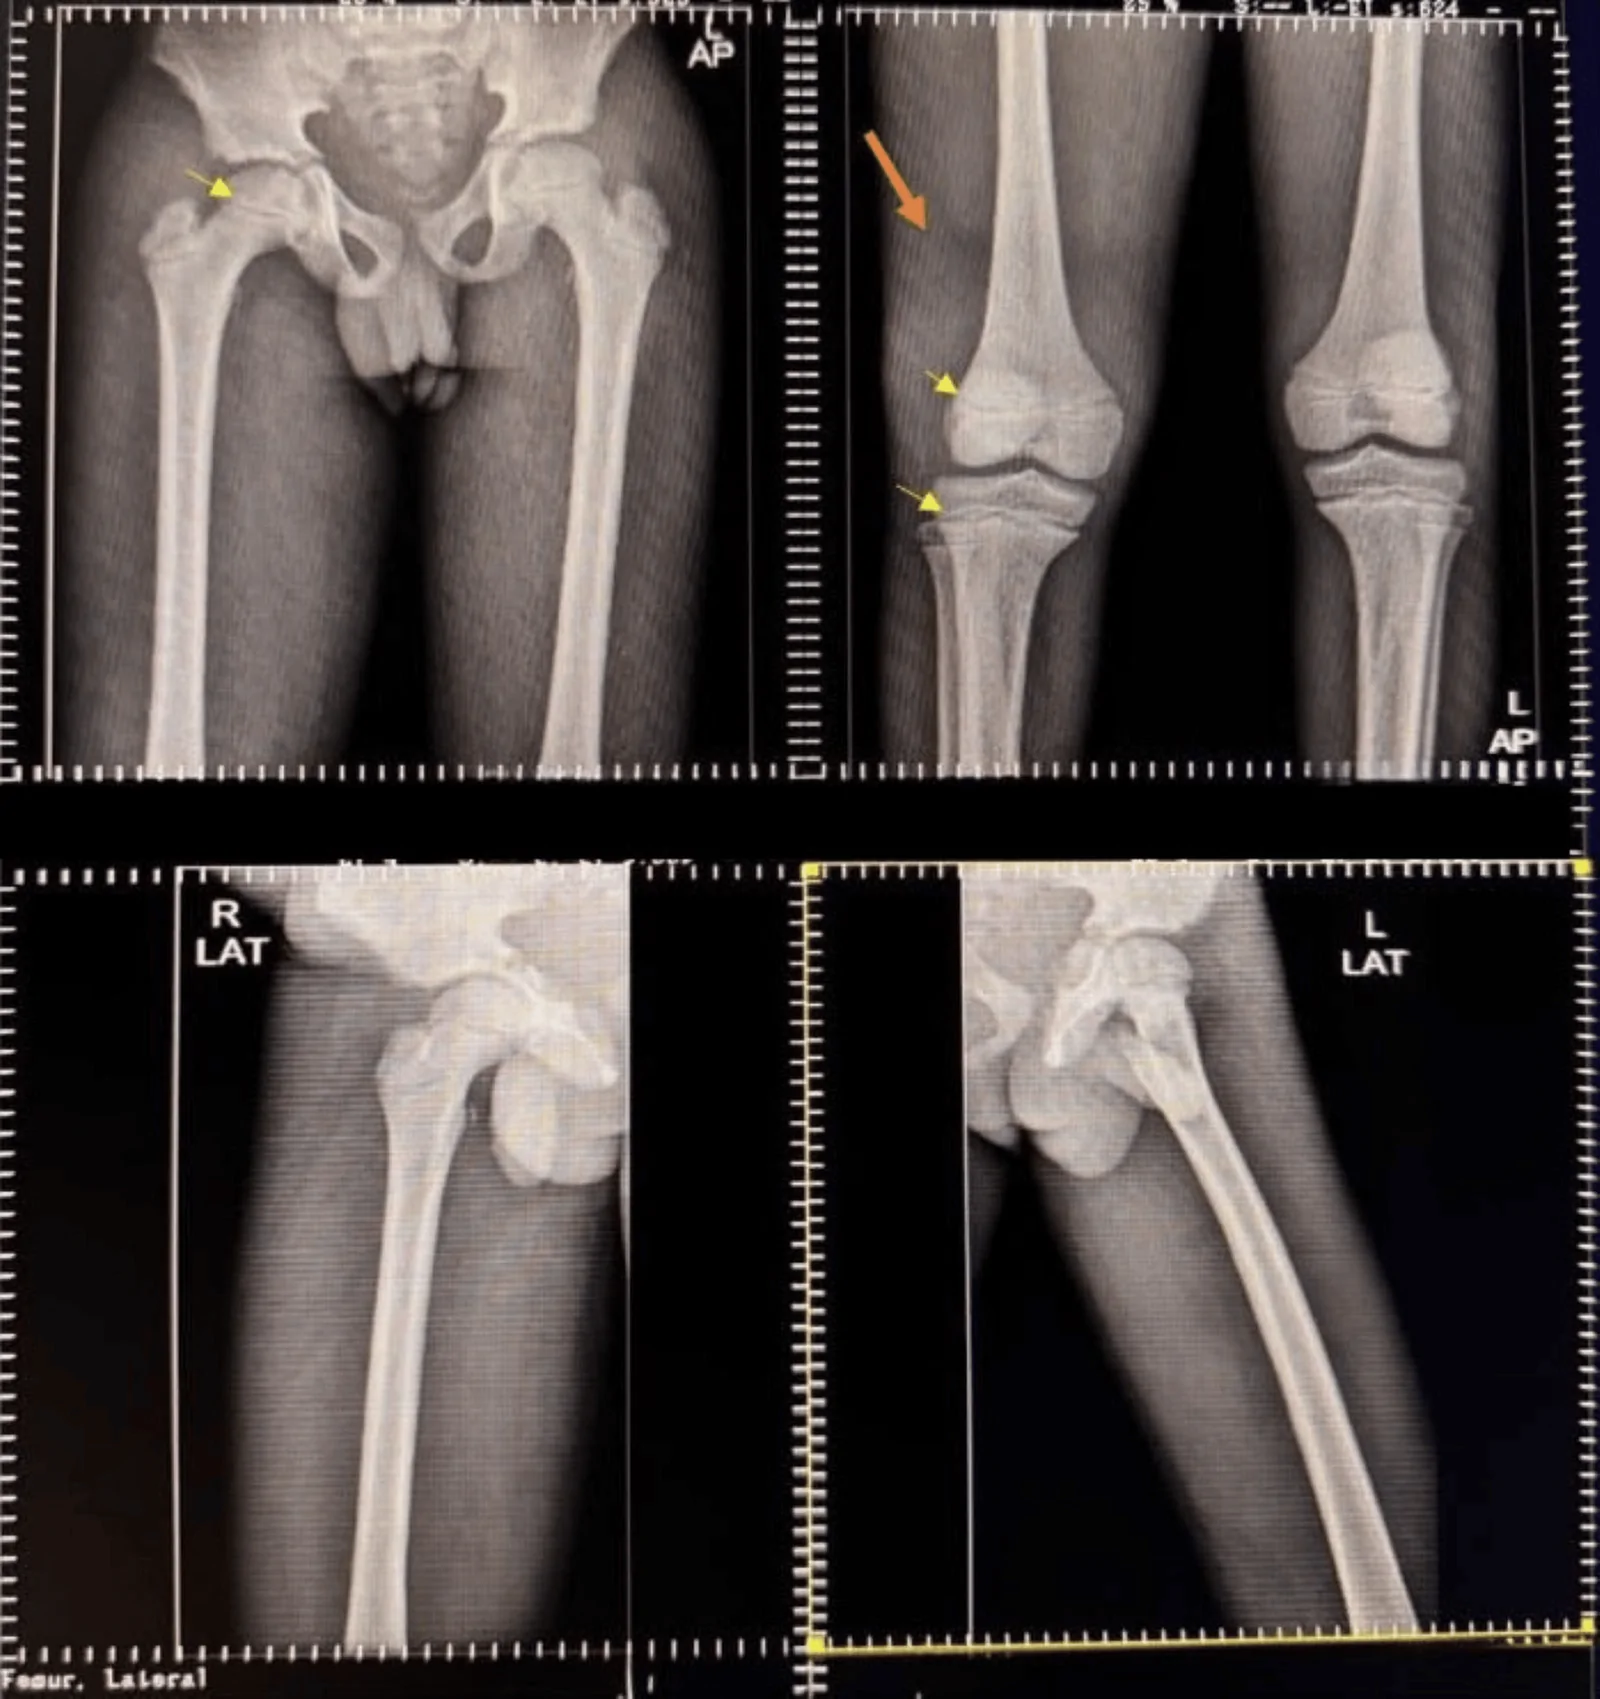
Plain radiograph of the knees and bilateral femora. Orange arrow: soft tissue swelling of the right thigh; yellow arrowhead: metaphyseal/physeal growth plates.
